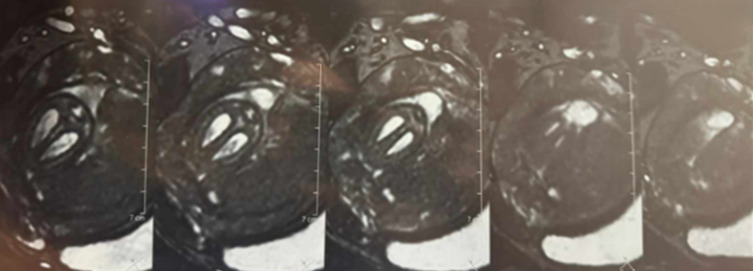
fetal MRI showing an occipital sub-torcular meningocele
